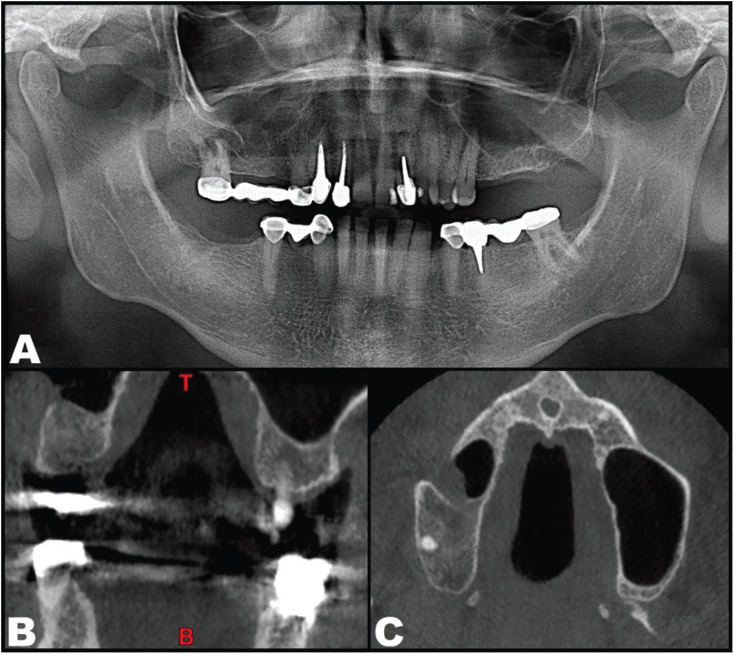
Radiographic control 12 months after surgery. A. Panoramic X-ray view. B. Cone-beam computed tomography coronal view. C. Cone-beam computed tomography axial view.
